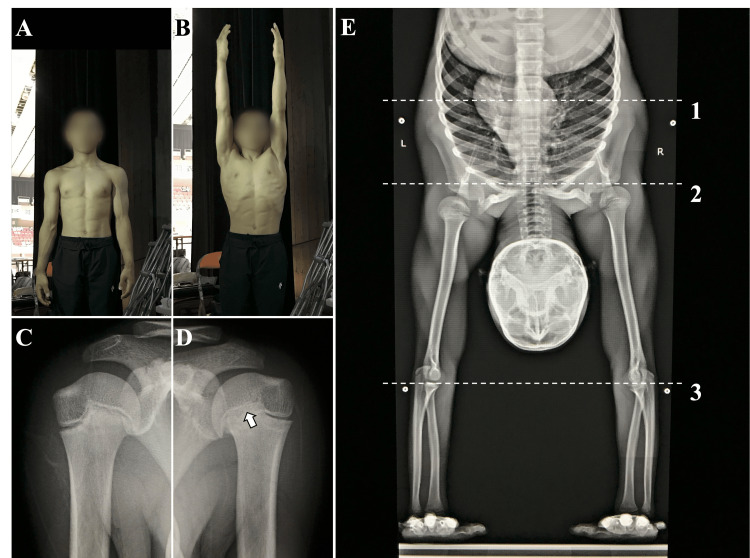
Case 2 A, B: When the arm was in a hanging position, the affected side was shorter in limb length. On the other hand, when the upper limb was raised, there seemed to be no difference in limb length on the left side. C: Radiograph of the healthy side. D: Radiograph revealed a closure of the medial portion of the proximal humeral physis compared to the healthy side  (arrows). E: A full limb radiograph in the handstand position. The humeral length was shortened, but the handstand was executed due to the compensatory movement of the scapula. Line 1: Height of the distal edge of the healthy side's scapula. Line 2: Height of the glenohumeral joint of the healthy side. Line 3: Height of the radioulnar joints on both sides.
